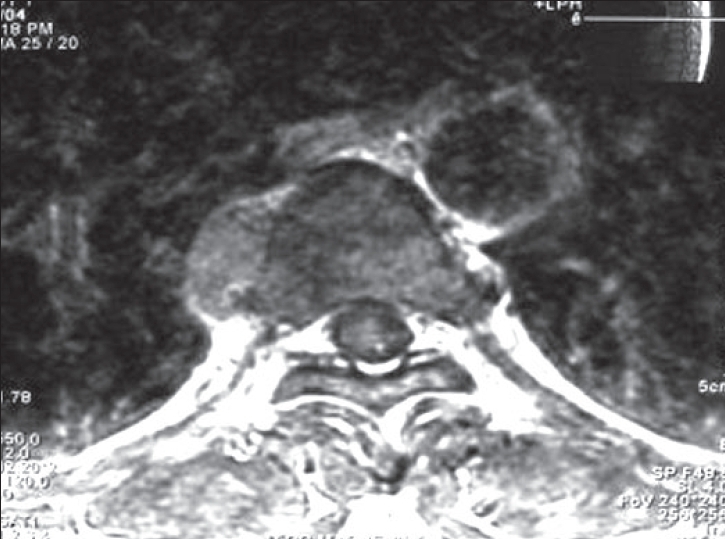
MRI showing a 1.5 × 3.0 cm mass along the lateral margin of the vertebral body. No extension into the neural foramen or spinal canal is demonstrated
